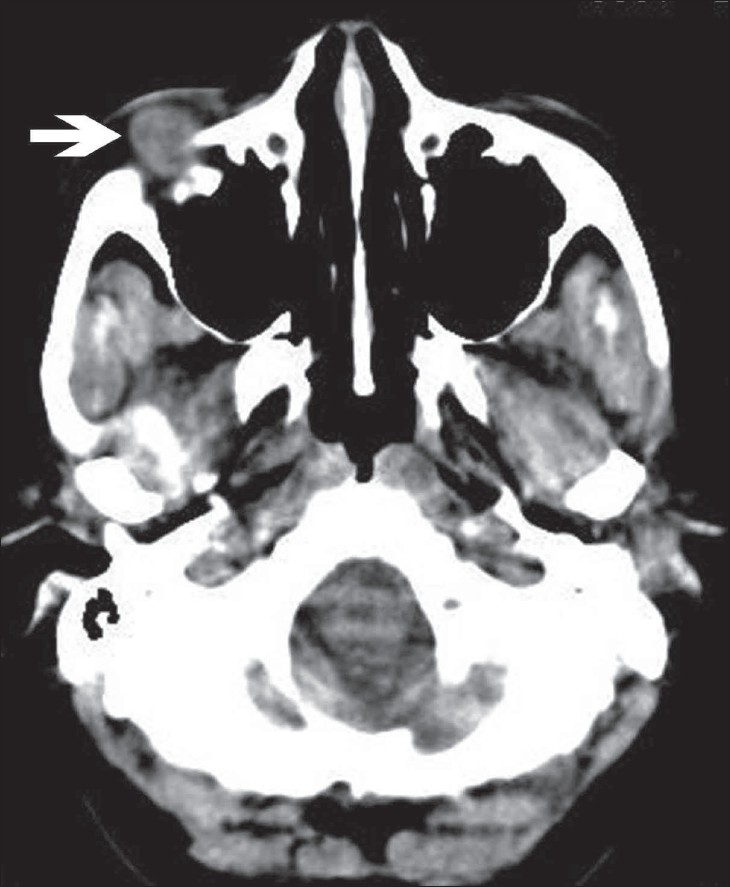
Plain axial CT image of the orbit showing a well-defined, heterogeneous lesion in the right orbit, extraconal in location (arrow)
